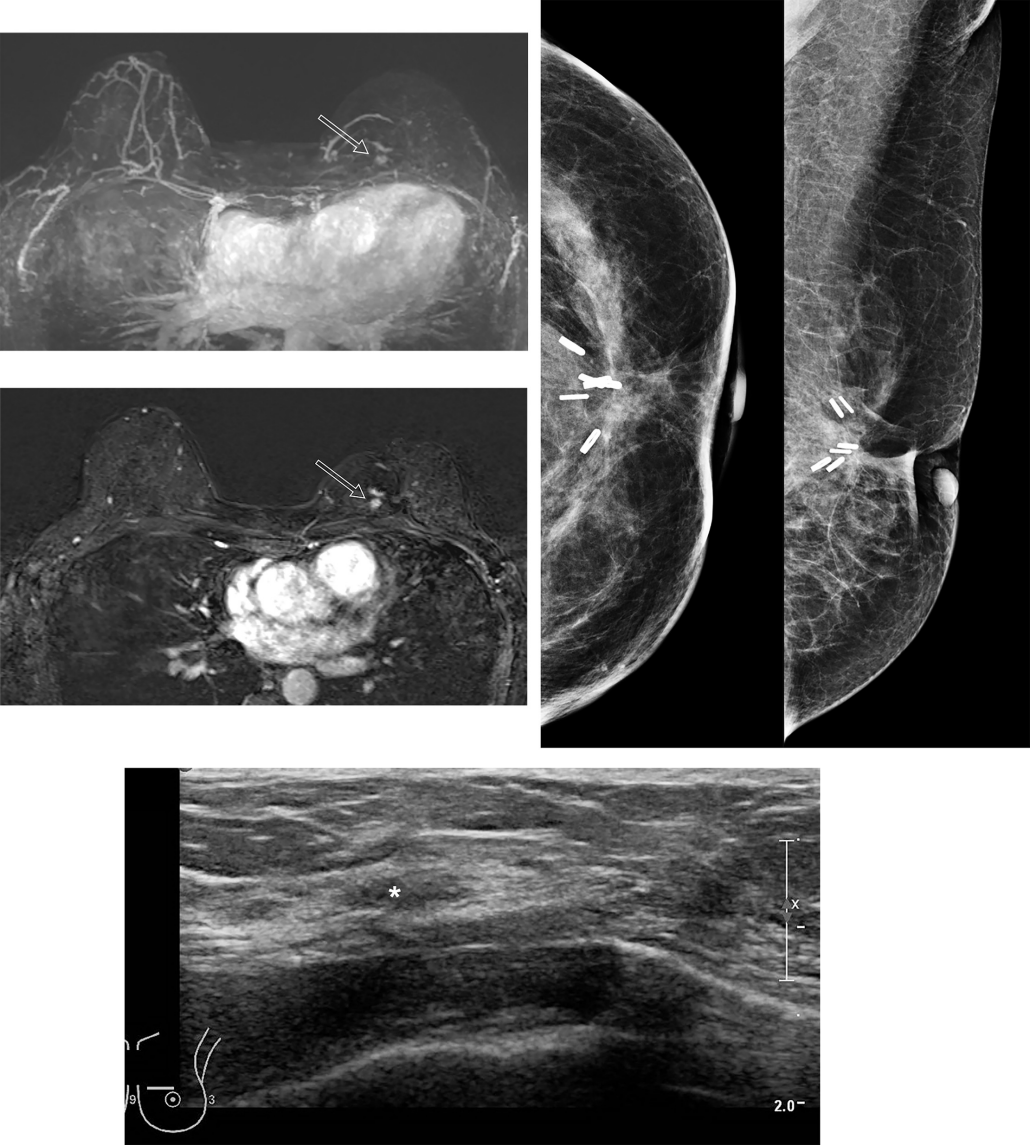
A 62-year-old female patient with an early recurrent breast cancer detected 12 months after BCT. (a) MIP image of postoperative MRI after 12 months from BCT for left breast cancer. There is a prominent nodular enhancement at upper inner quadrant of left breast (open arrow). (b) On subtracted early dynamic phase of contrast enhanced T1WI, a brightly enhancing nodule is noted adjacent to previous BCT site (open arrow). (c) There is no abnormal finding on mammography of the same day as breast MRI. (d) Second look ultrasound for biopsy shows oval parallel, indistinct isoechoic lesion (white star) mimicking normal parenchymal pattern at corresponding site of enhancing lesion detected on MRI. This lesion was not detected on breast ultrasound performed at the same day as breast MRI.
